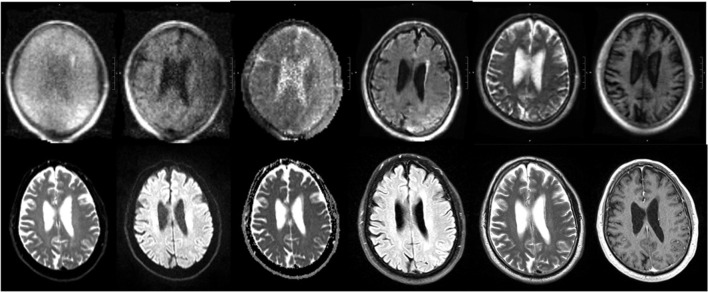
pMRI vs. conventional MRI. Sample pMRI images (top row) are provided along with the corresponding conventional imaging (bottom row). The sequences from left to right are: DWI (*b* = 0), DWI, DWI with ADC map, FLAIR, T2W, and T1W.
